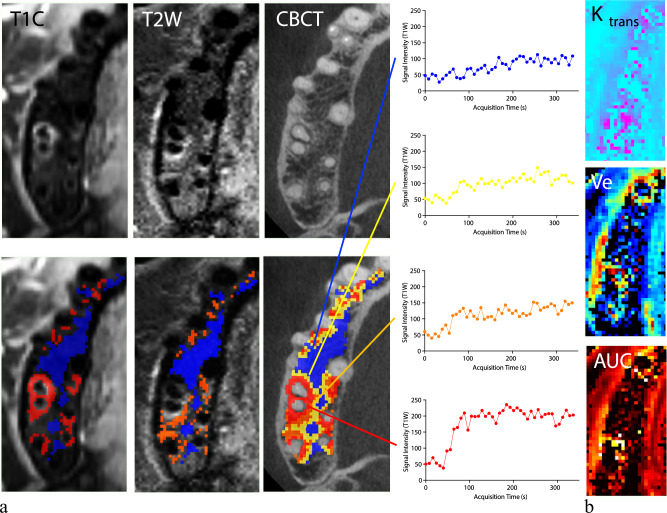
Dynamic contrast-enhanced perfusion imaging analysis. a T1-weighted contrast-enhanced images (T1C), T2-weighted images (T2W), and cone-beam computed tomography (CBCT) images to demonstrate the segmentation of lesions based on distinct imaging characteristics. The segmentation differentiates between periodontal lesions showing increased signals in T1C imaging (T1C+, highlighted in red), areas being hyperintense on T2W but not enhancing on T1C (T2W+, marked in orange), perilesional areas appearing normal in both T1C and T2W (PL, yellow), and the normal bone marrow distant from the lesions used as control (C, indicated in blue). The corresponding time-intensity curves depict a faster and greater uptake of the contrast agent in areas impacted by periodontitis relative to the distant bone marrow that appears normal. b Corresponding maps for the volume transfer constant (K_trans_), the extravascular extracellular space volume fraction (V*e*) and the area under the time-signal intensity curve (AUC)
